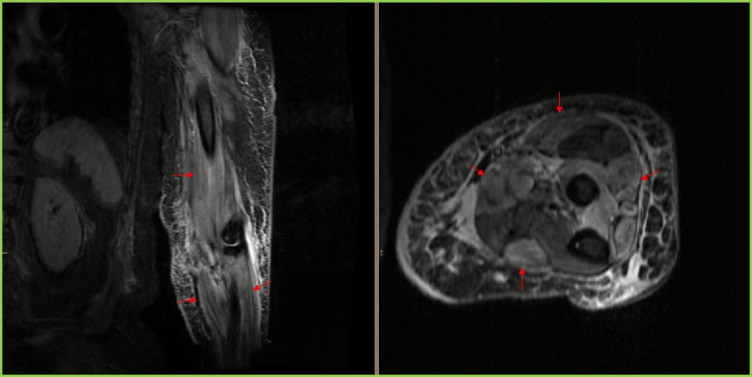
A, B Coronal STIR image of the left arm and axial T2-weighted image with fat saturation of the left forearm. Coronal STIR image of the left arm and axial T2-weighted image. There is diffuse, circumferential subcutaneous edema and increased signal of muscles, with geographic pattern of involvement of multiple compartments (arrows). Imaging findings consistent with myositis post vaccination on the left arm against SARS-CoV-2 virus
